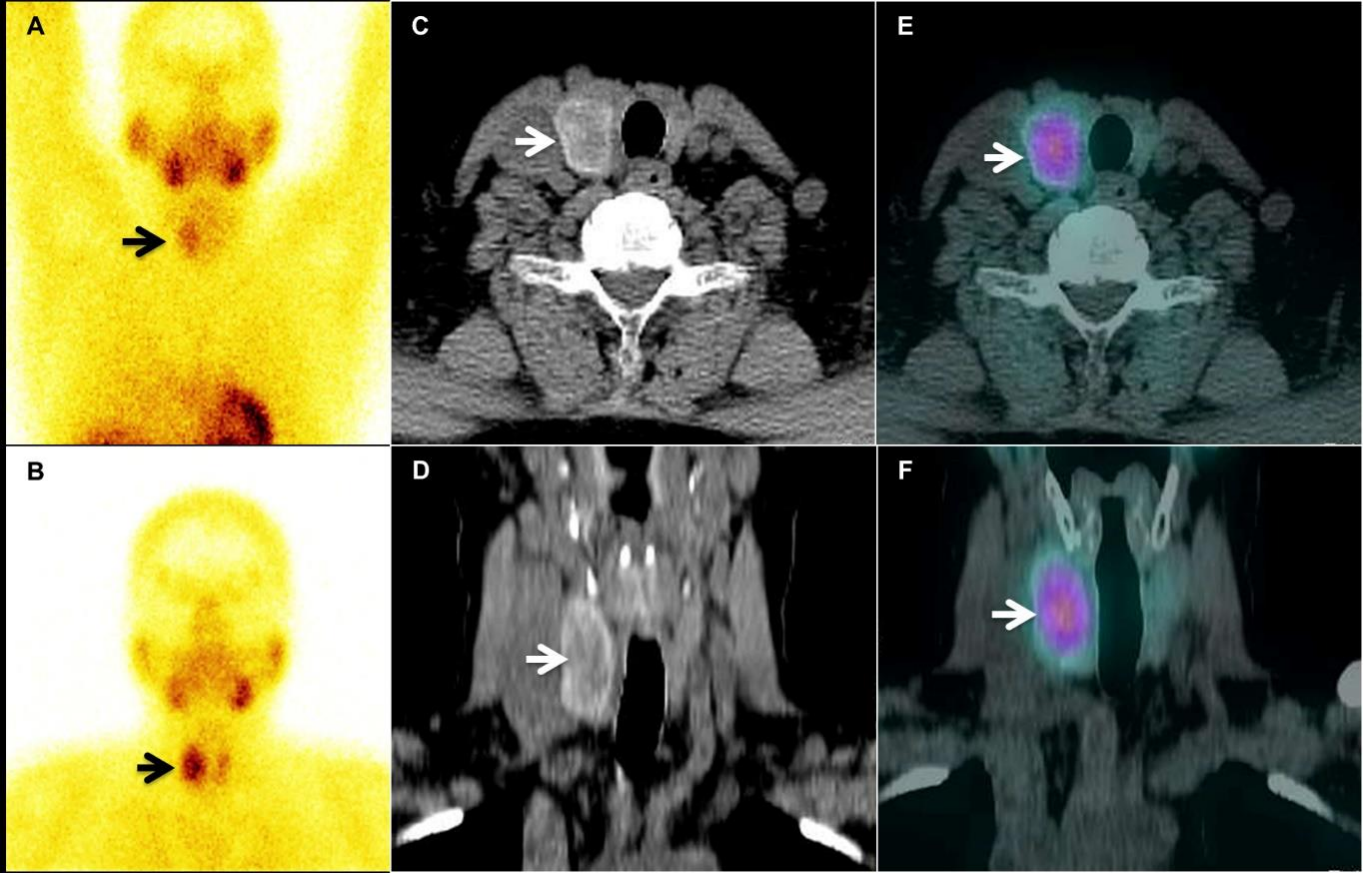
The early-phase planar static image acquired 10 minutes post-injection of 20 mCi (~740 MBq) of Tc-99m sestamibi (A) revealed a focus of relatively increased tracer uptake in the right lobe of the thyroid gland with differentially lower washout compared to the rest of the gland on the delayed static image at 1 hour (B). The early SPECT/CT images localized the tracer activity to a heterogeneous hypodense lesion within the right lobe of the thyroid gland on the axial and coronal CT (C,D) and corresponding fused SPECT/CT images (E,F). There were areas of central cystic/necrotic changes within the lesion on the CT images with no other abnormal tracer avid lesion elsewhere in the neck or the mediastinum
